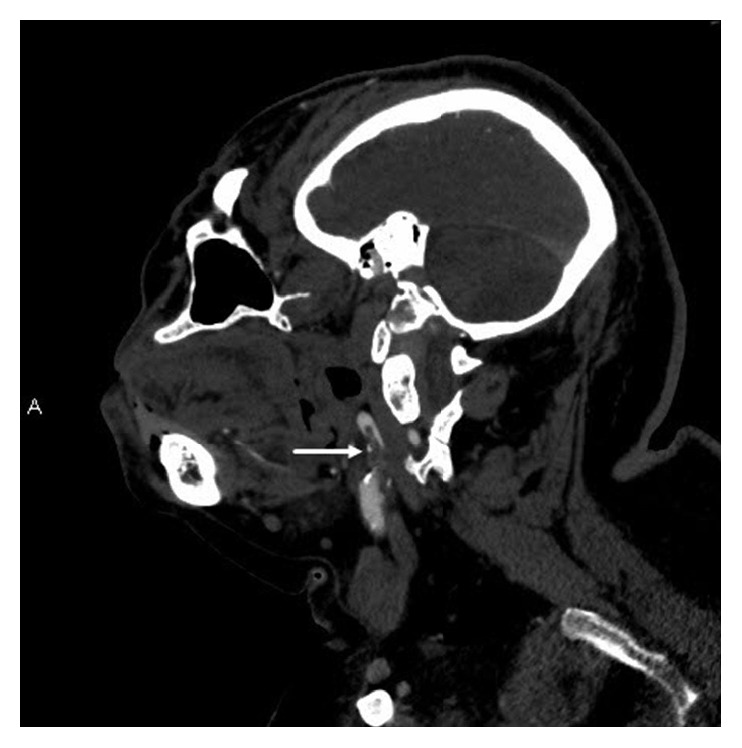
Sagittal oblique reformatted view from CT angiogram again depicts the intraluminal thrombus within the left internal carotid artery (white arrow).
